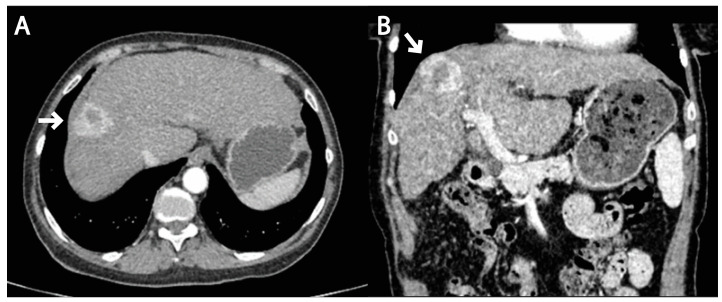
Axial (A) and sagittal (B) images of a contrast-enhanced CT scan obtained in the arterial phase reveal the presence of a solitary, hypervascular liver tumor in segment VIII of the liver (arrows), with washout in portal venous phase (not shown in these images), consistent with the radiological features of an HCC in the setting of a cirrhotic liver.
